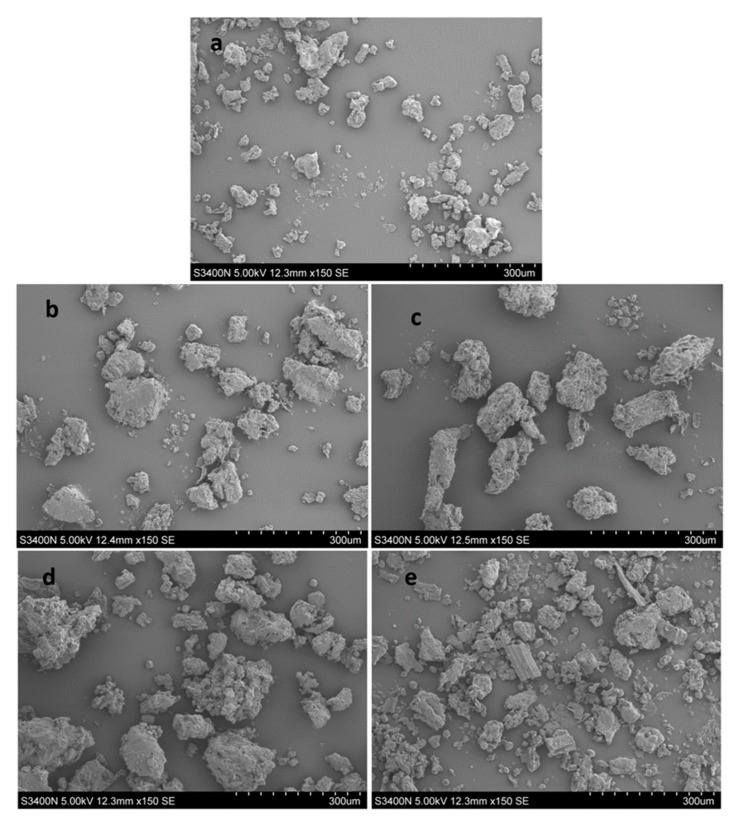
The SEM photos of (a) the unextracted crude drug powder, (b) ultrasonic-assisted methanol extraction with enzyme pretreatment, (c) ultrasonic-assisted 70% ethanol extraction with enzyme pretreatment, (d) ultrasonic-assisted DESs extraction and (e) ultrasonic-assisted DES extraction with enzyme pretreatment.
